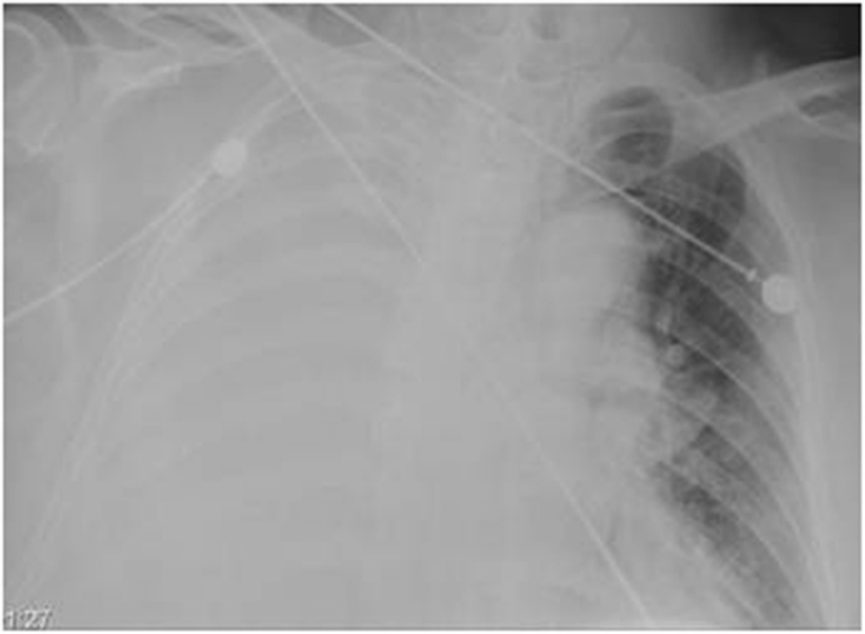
Chest radiograph showing a whole consolidation and/or pleural effusion of the right lung.
